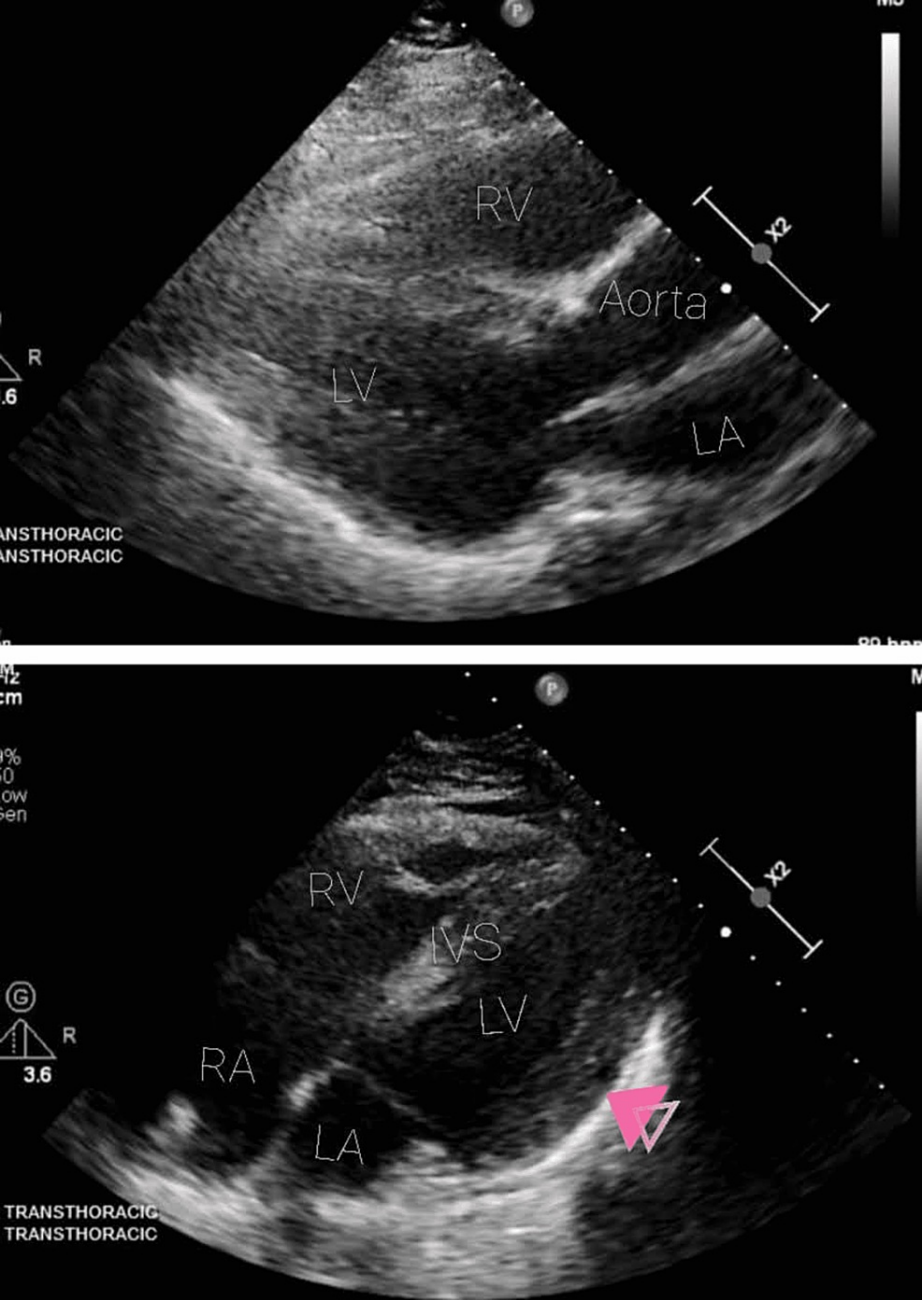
Transthoracic echocardiogram Top image: parasternal long-axis view of the heart. Bottom image: apical four-chamber view of the heart. Arrowhead indicates left ventricular hypertrophy (LVH). LA - left atrium; RA- right atrium; LV - left ventricle; RV - right ventricle; IVS - interventricular septum
